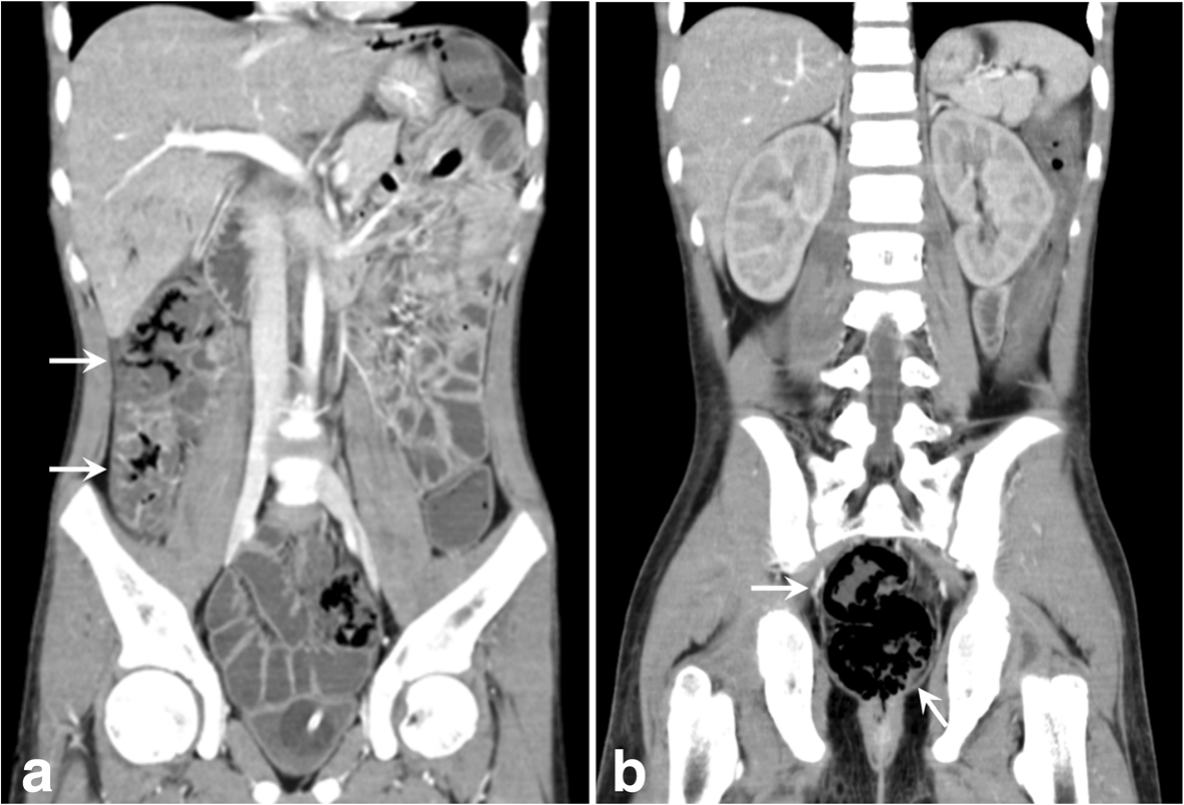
Contrast-enhanced abdominal computed tomography (CT). Emergency CT scans revealed pneumatosis intestinalis (arrows) localized in the ascending colon (a) and the rectum (b) coexisting with portal venous gas
